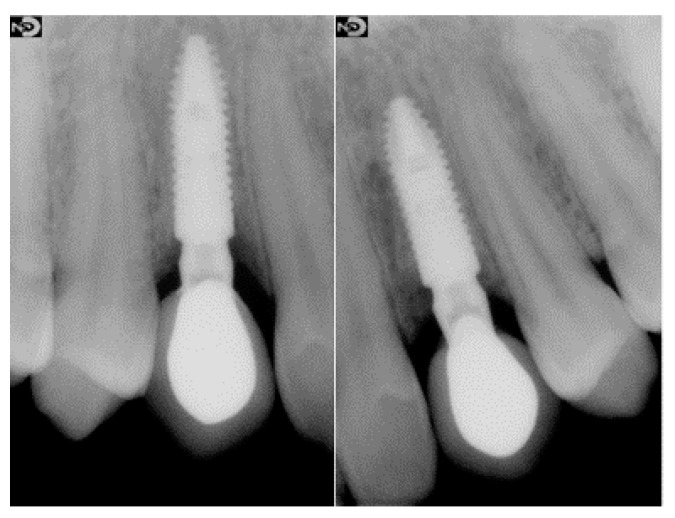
Radiographs of definitive restorations after 2-year follow-up.
